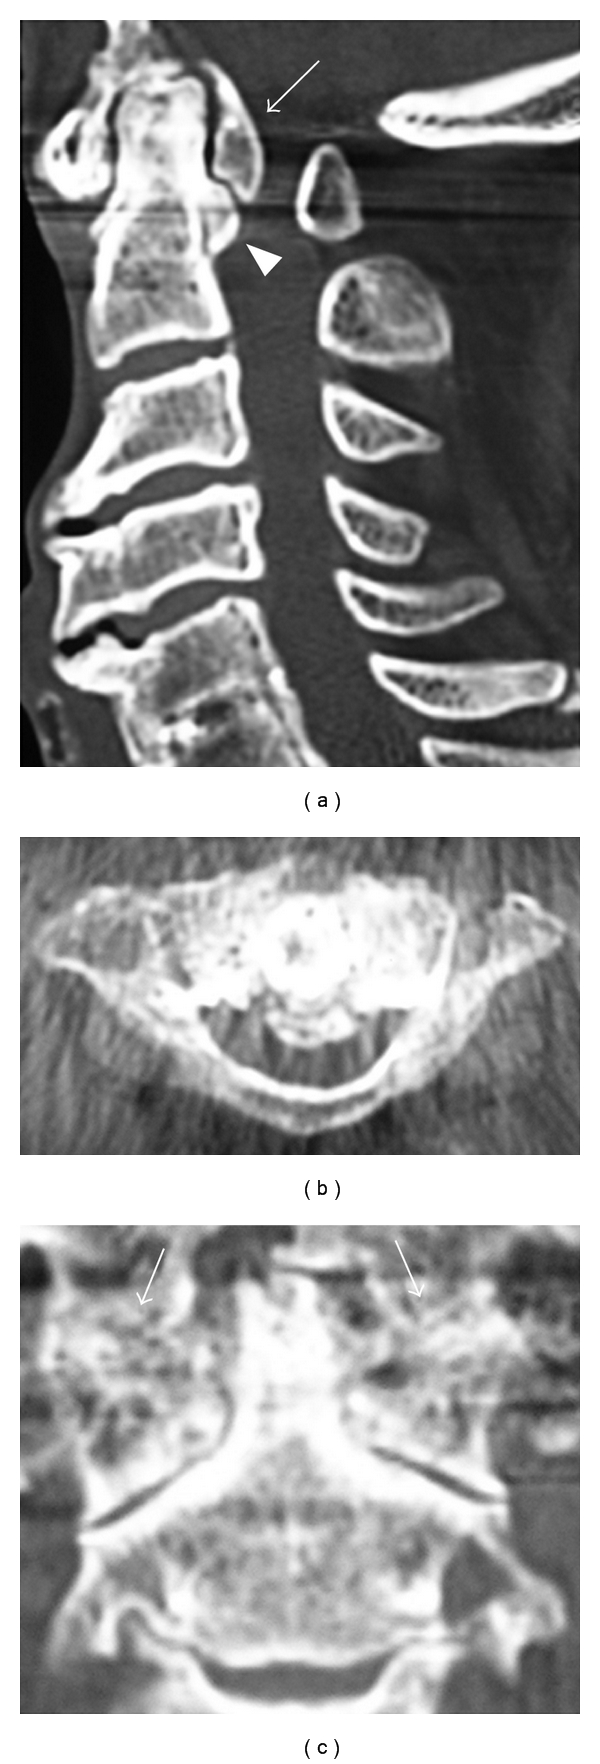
Reconstructed computed tomogram (CT). (a) Sagittal reconstructed CT. Ossified mass behind dens (arrow) and osteophyte continuing from the dens (arrowhead) can be seen. The dens itself is degenerated and hypertrophied. (b) Axial CT. Most of the spinal canal is occupied by ossification of transverse ligament of atlas. (c) Coronal reconstructed CT. The joint space of atlantoaxial joint and the spaces between dens and lateral masses of atlas were narrowed. Coalition of bilateral atlantooccipital joints can be seen (arrow).
